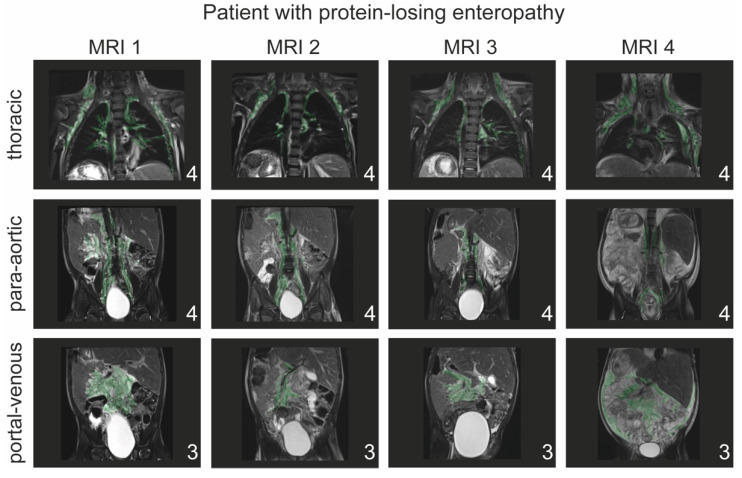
Example of patient with protein-losing enteropathy. Patient who presented with prolonged pleural effusion (28 days) and edema after TCPC requiring diuretic therapy. Over the next two years, the patient presented with continuous clinical deterioration requiring frequent admissions to hospital for volume overload, recurrent pleural effusion, diarrhea and protein loss. The patient underwent serial cardiac MRI and cardiac catheter studies targeting hemodynamic parameters. Despite clinical variation in the presentation, lymphatic classification did not change during the entire period. A lymphatic fistula to the left lower lobe bronchus and thoracic cavity can be visualized at MRI 1 and remains visible at all four MRIs. At the time of MRI 4 the patient presented with clinical deterioration and a pleural effusion on the left side and ascites in addition to the same degree of lymphatic abnormalities. Lymphatic abnormalities are highlighted in green.
